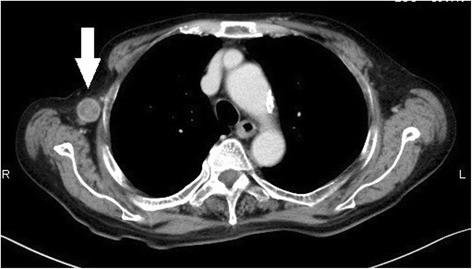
CT demonstrating an enlarged lymph node of 3 cm in diameter in the right axillary region (*white arrow*)
